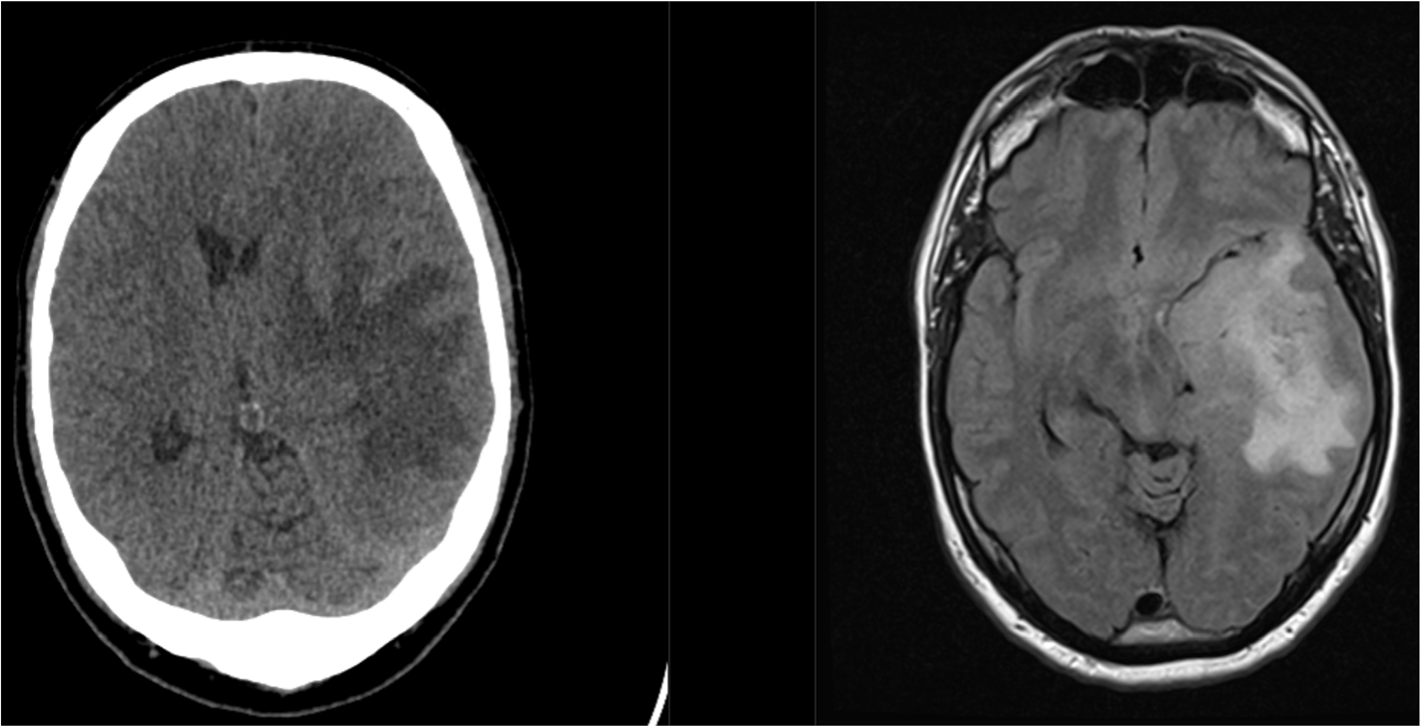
6 months after pacemaker implantation; CT (left) and MRI of newly diagnosed glioblastoma
